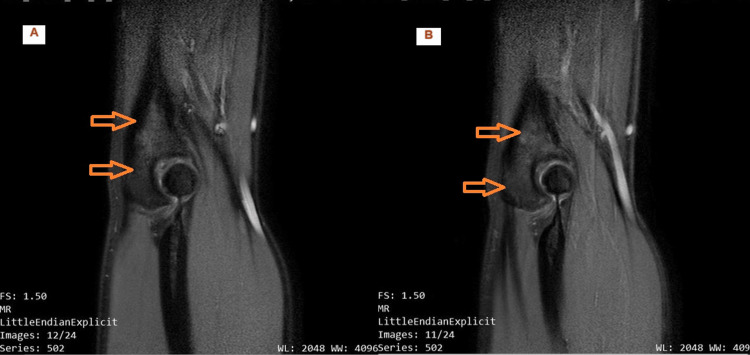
Two sequential sagittal images on first MRI. (A and B) Diffuse bone marrow oedema of distal humerus two months after the onset of symptoms.
